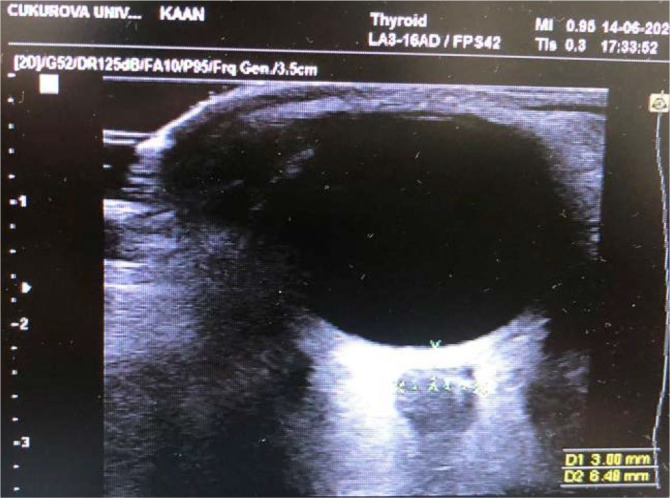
Measurement of optic nerve sheath diameter of our patient by ultrasonography
